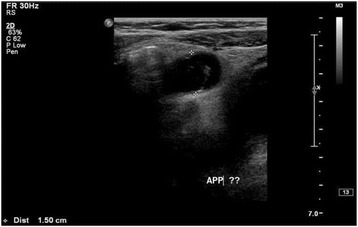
Ultrasound abdomen for 5 years old boy presented with abdominal pain showed an 1.5 cm non compressible tubular-like structure suggestive of appendicitis
